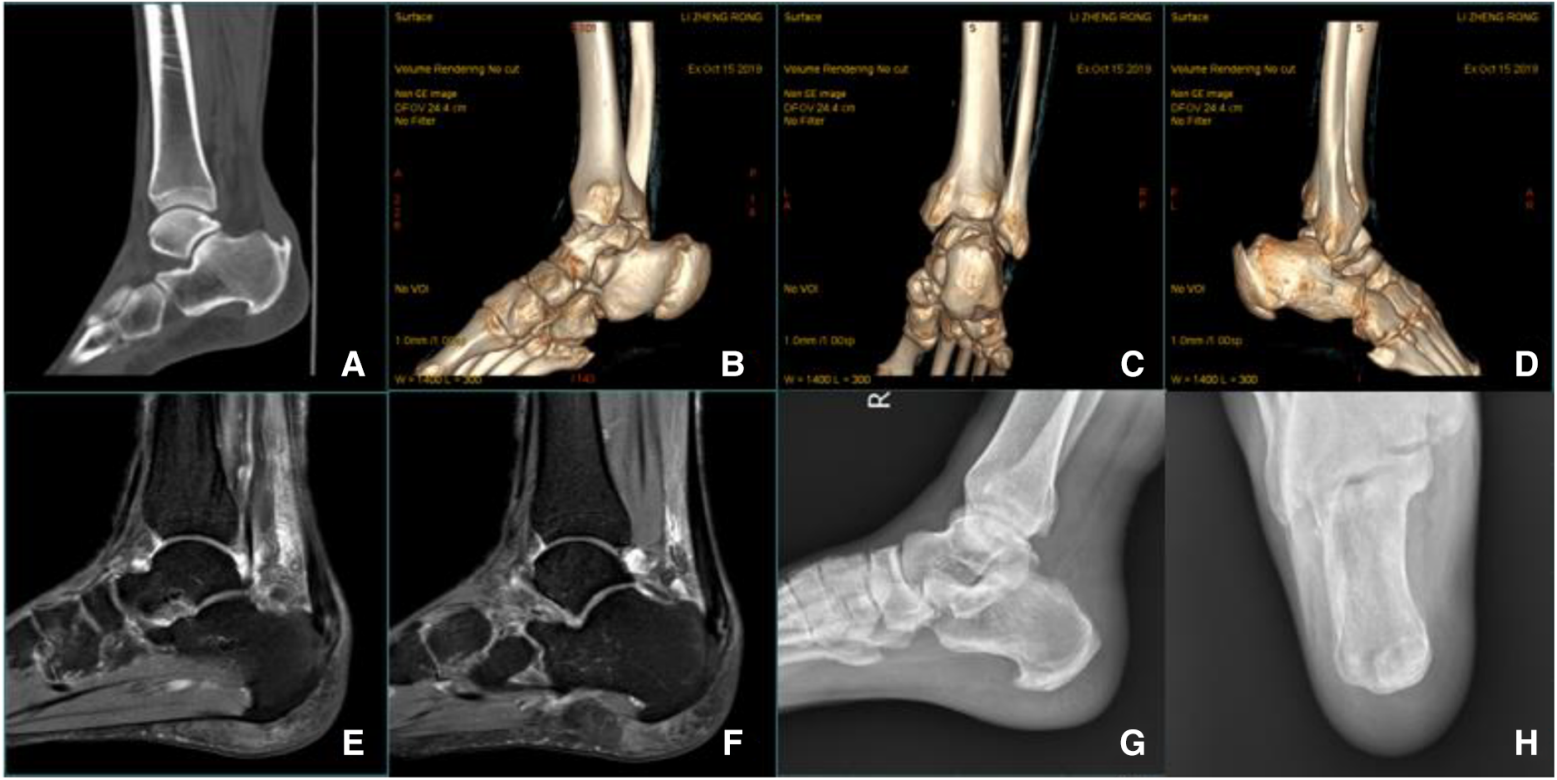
(A–D) Preoperative CT scan: Calcification of Achilles tendon insertion was evident, accompanied by Haglund deformity. (E, F) Preoperative MR imaging: Denaturation and calcification at the insertion of the Achilles tendon, fluid accumulation behind the ankle joint, and compression of the flexor hallucis longus tendon. (G, H) Postoperative radiography: The posterior calcaneus was flat, and there was no Haglund deformity at the insertion of the Achilles tendon.
